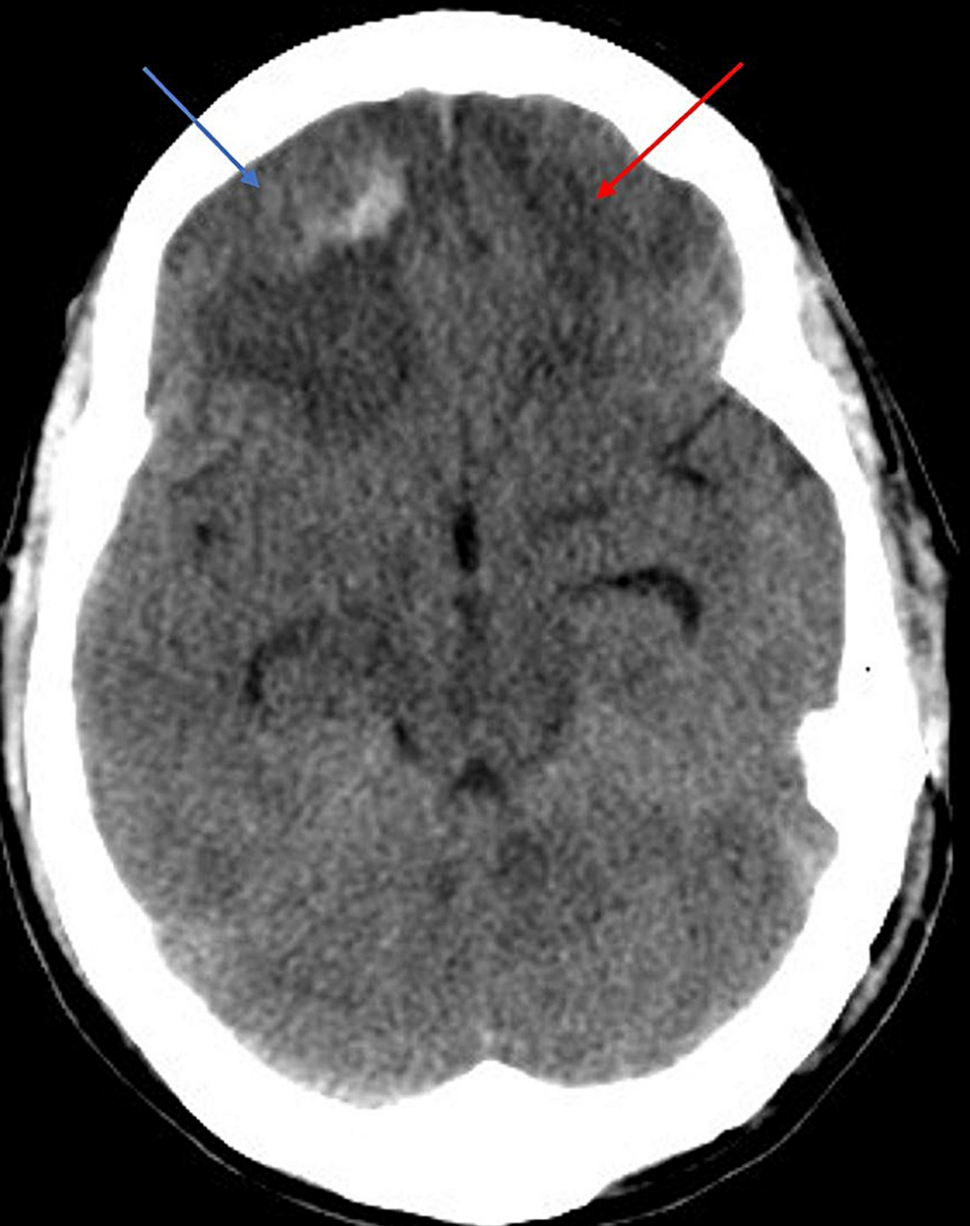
CT head without contrast at discharge showing resolving traumatic intracranial hemorrhages Blue arrow: resolving right frontal sub-dural hematoma, red arrow: resolving left frontal hemorrhagic contusion
